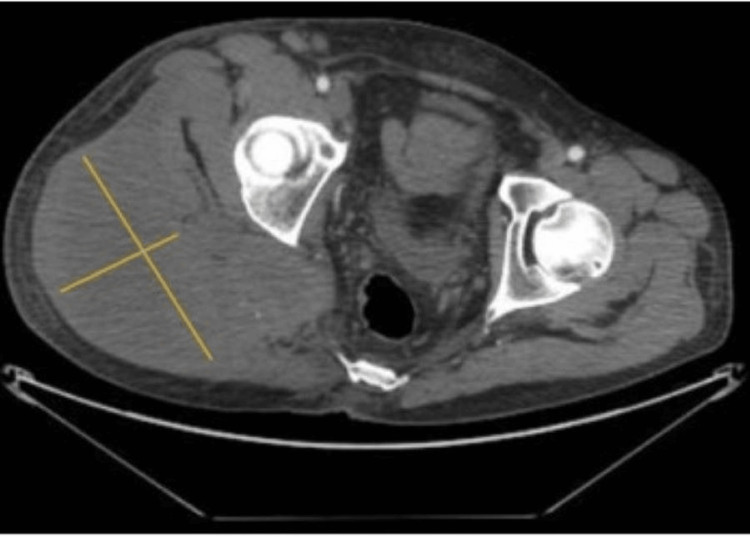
CT abdomen CT abdomen showing a large, irregular, necrotic right gluteal mass
